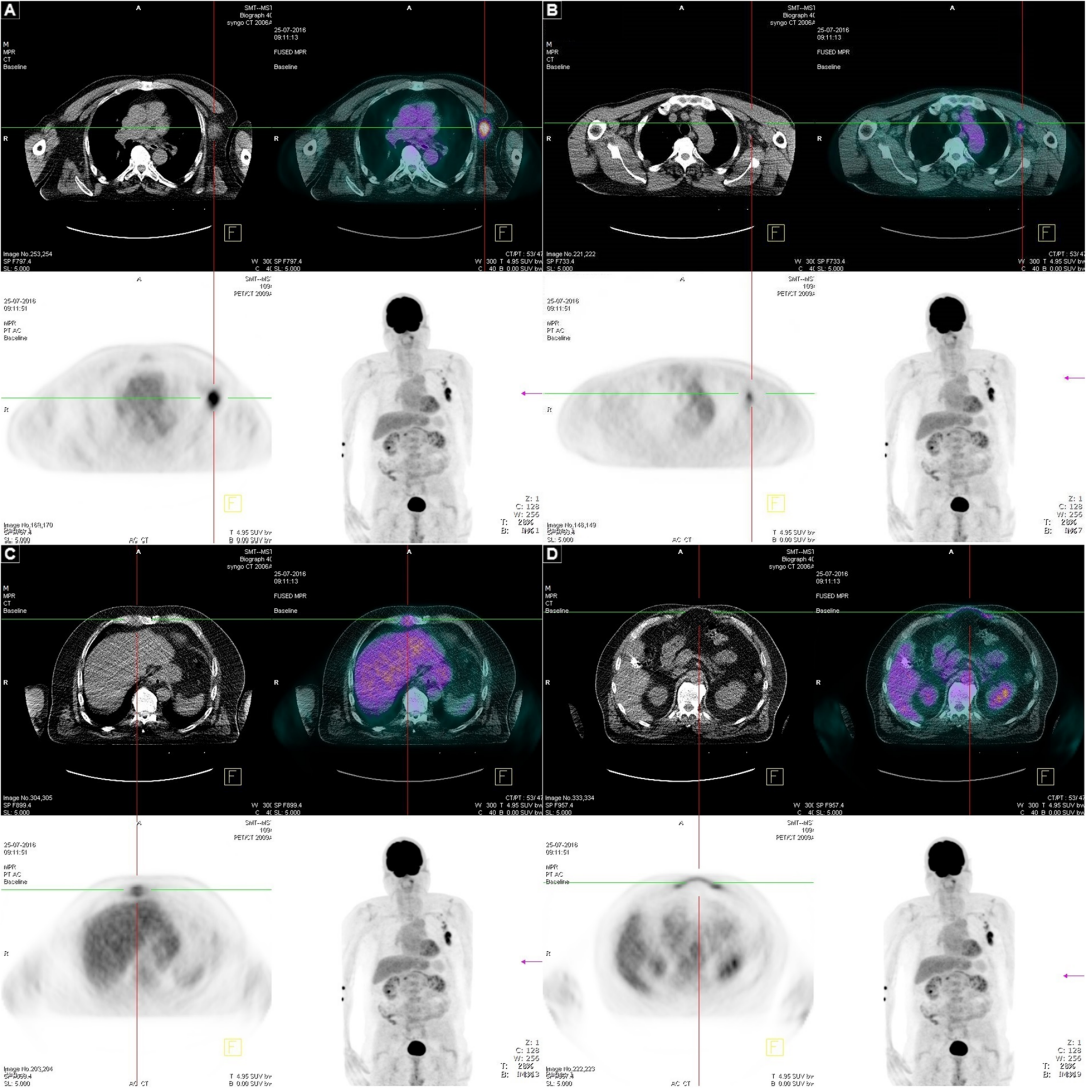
Positron emission tomography–computed tomography of the metastases that occurred almost 6 years after initial cholecystectomy. PET-CT (from the bottom right, clockwise) of the upper body, the axial PET, low-dose CT, and fused PET-CT. a axillary metastasis, b subpectoral uptake, c right parasternal mass, and d epigastric port site scar
